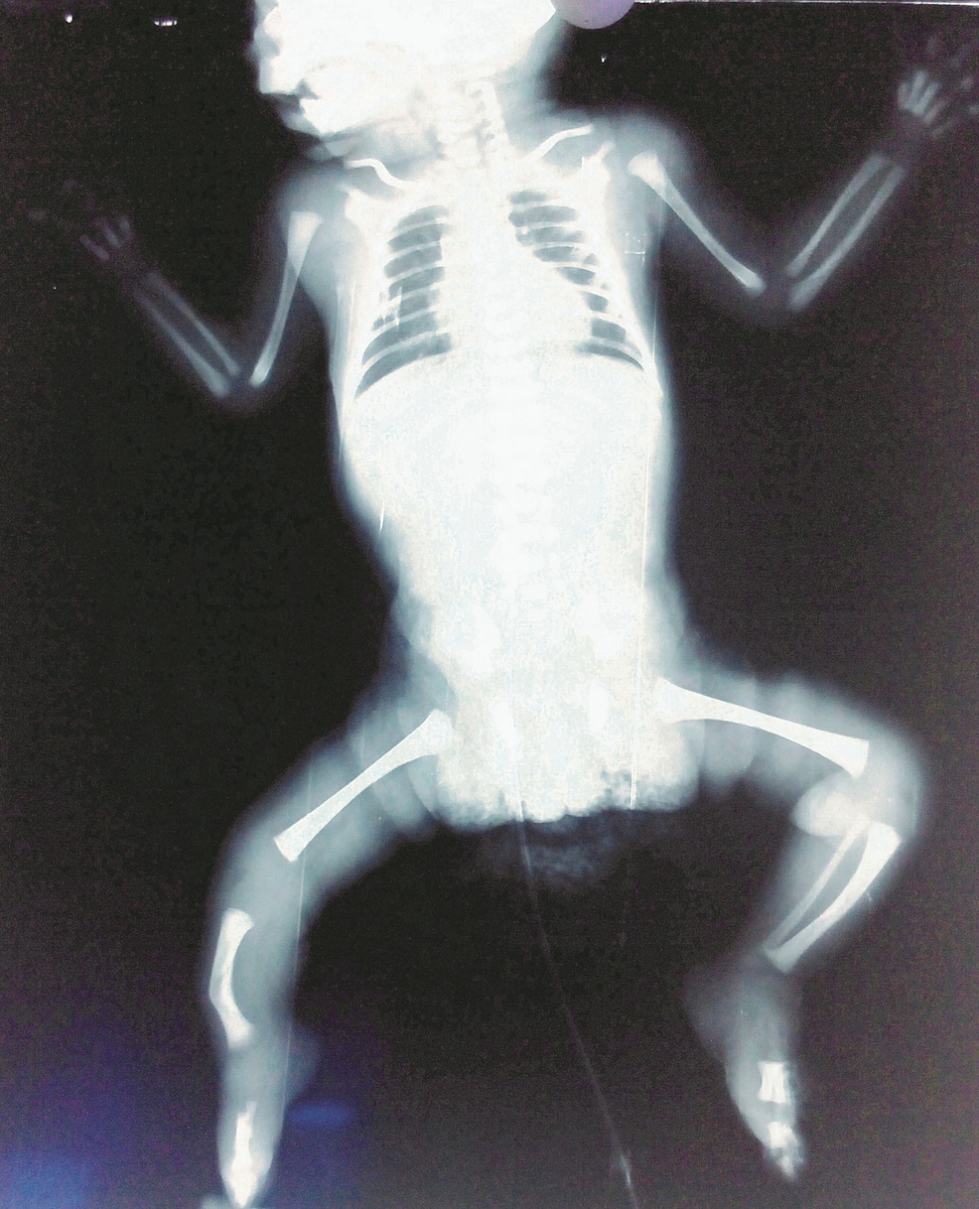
Radiograph of the patient. It shows the right hand with only three metacarpals, the normal left hand, right fibular hemimelia, middle tibial campomelia, and absent calcaneus and talus ossification centers and normal femurs, left tibia, and left fibula.
